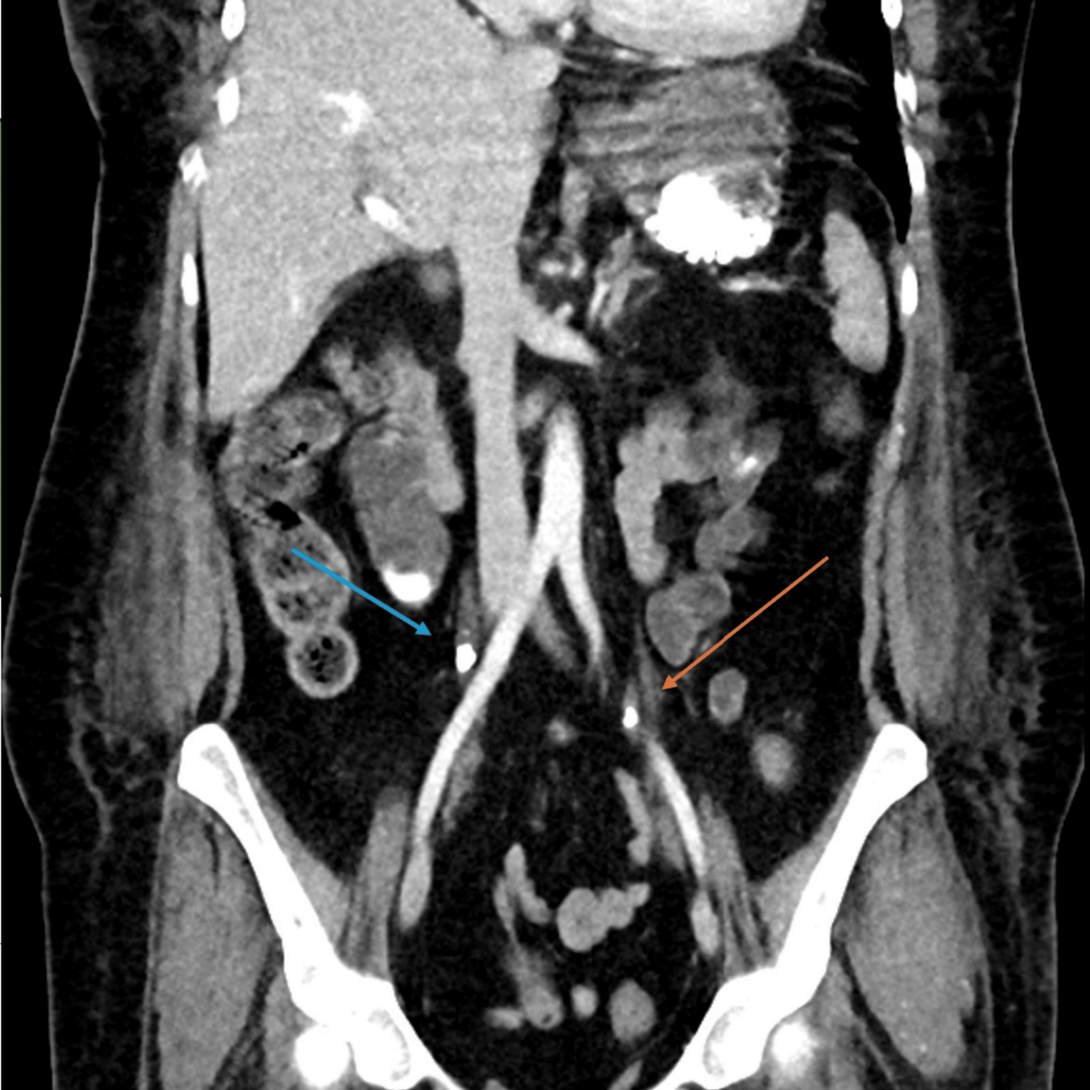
CT abdomen/pelvis sagittal view demonstrating bilateral nephrolithiasis, highlighting a 0.9cm stone in the left ureter (orange arrow) and a 1.0 cm stone in the right ureter (blue arrow) along with associated bilateral moderate hydronephrosis.
